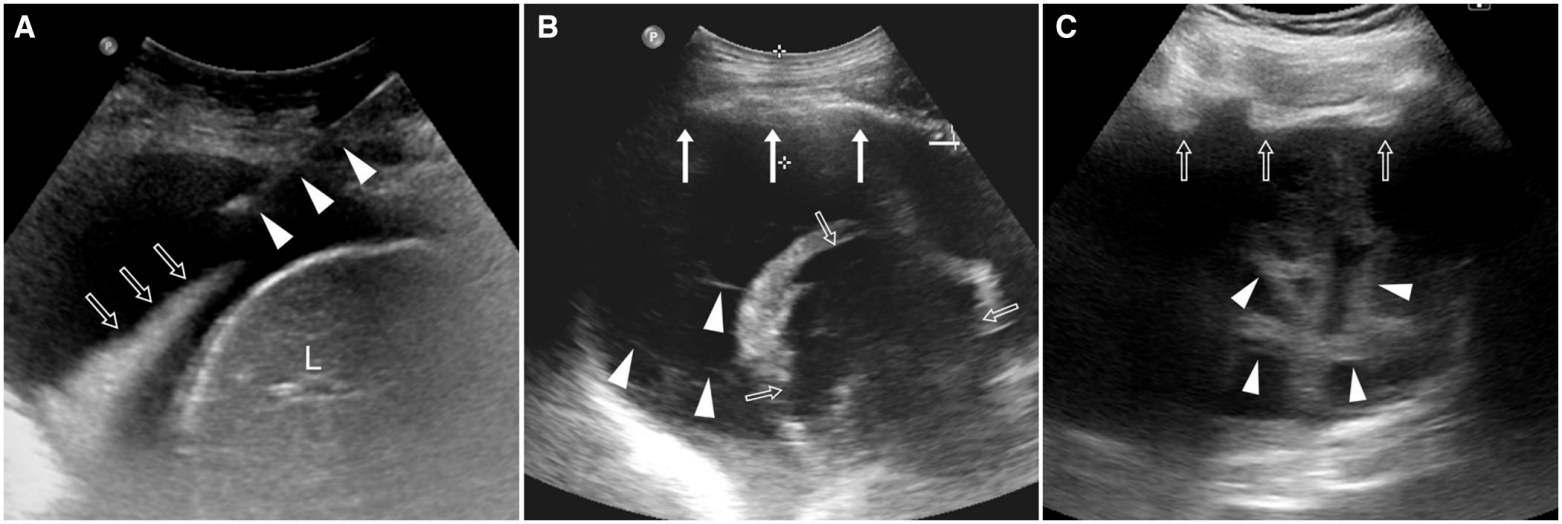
Various appearances of ultrasound (US) imaging in patients with pleural TB. All patients were examined with convex US transducers scanning through the intercostal space. (A) US image in an 85-year-old woman shows homogeneously anechoic effusion with underlying lung atelectasis (open arrows). US-guided thoracentesis was performed (arrowheads indicate needle). L = liver. (B) US image in a 59-year-old man shows smooth pleural thickening (solid arrows) and complex pleural effusion with thin septa (arrowheads). There is also cavitation (open arrows) in the adjacent lung consolidation. (C) US image in a 60-year-old man shows irregular pleural thickening and nodularity (open arrows) with complex thick septations (arrowheads) within the pleural effusion.
